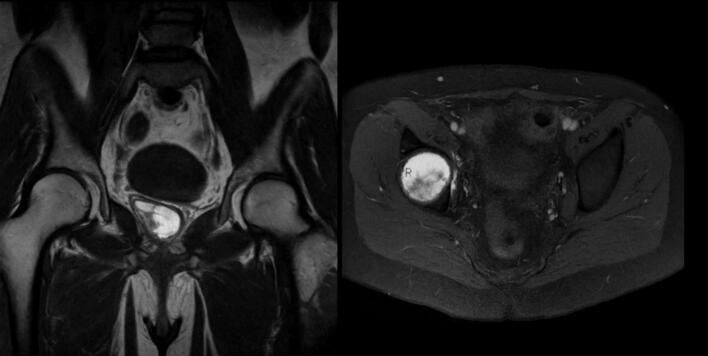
Pre-operative MRI of the patients coronal and axial view of patient IV.
